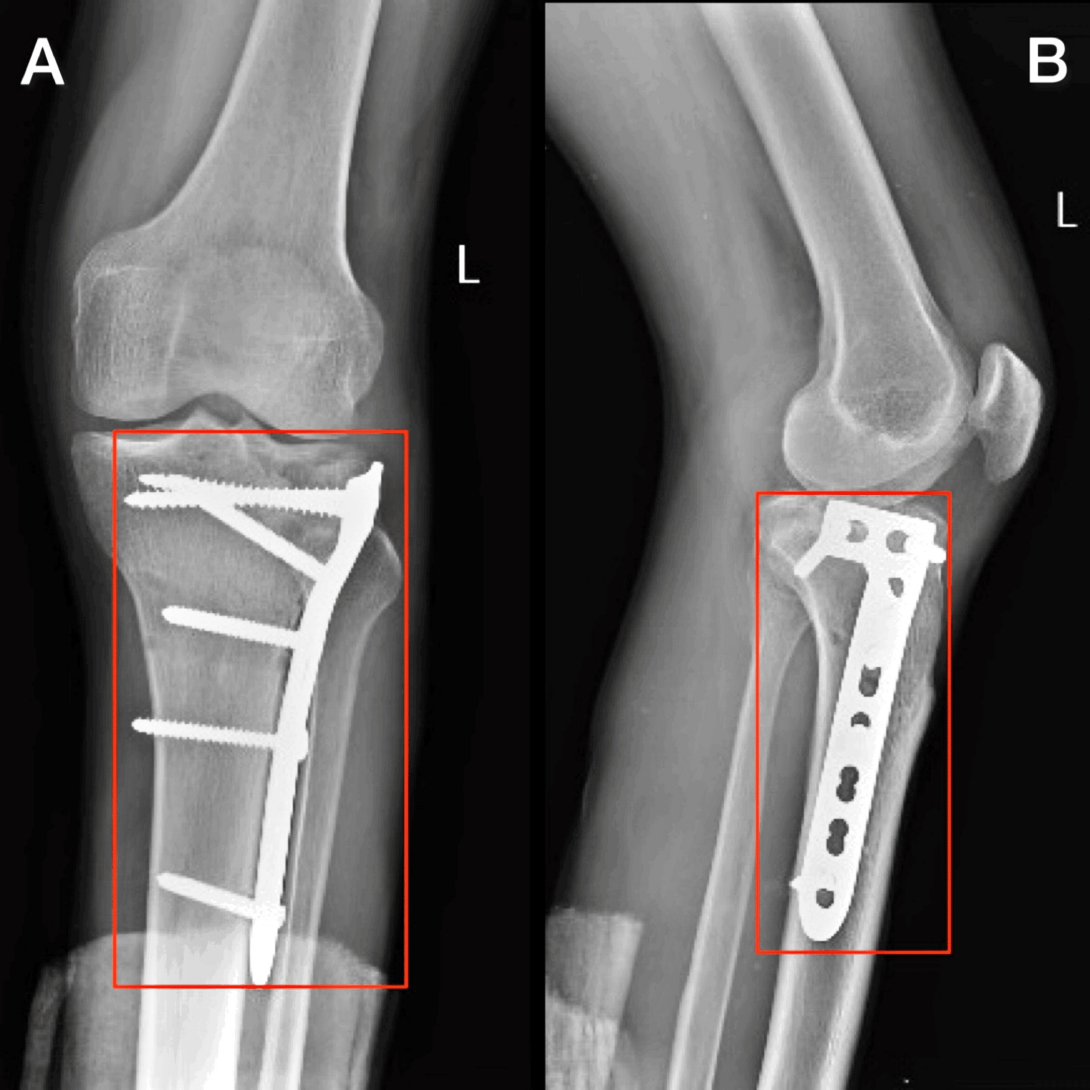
Postoperative X-ray of the left knee joint AP - antero-posterior A: AP view of knee joint showing cancellous screw and locking screw; B) Lateral view of ankle joint showing L-butress plate
